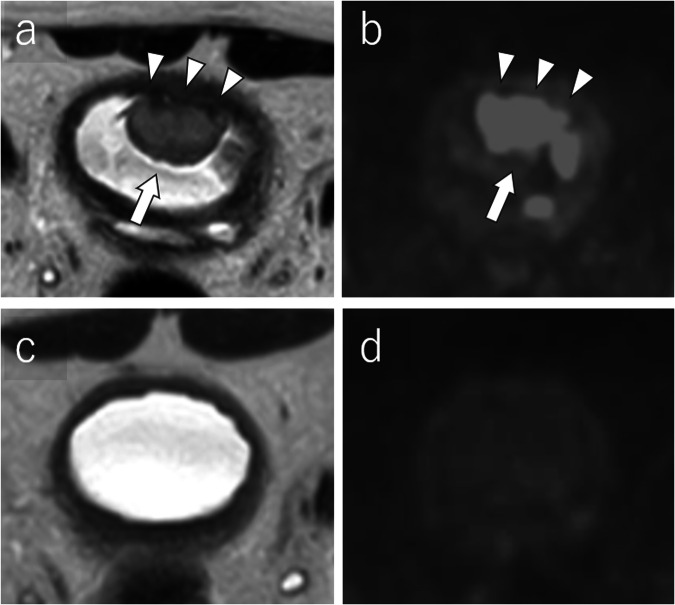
Immunotherapy responder: a 76-year-old man with a 35-mm urothelial carcinoma in the anterior bladder wall pre-immunotherapy MRI shows a round-shaped tumor with no apparent muscularis disruption on T2WI (a) [T2WI VIRADS score: 3] and homogeneous high signal on DW-MRI (b) [DW VI-RADS score: 3]. Post-immunotherapy MRI reveals significant tumor reduction, with no apparent lesions on T2WI (c) or DW-MRI (d). The tumor is indicated by white arrow, and intact muscle layers by white arrowheads. ADC, apparent diffusion coefficient; DCE, dynamic contrast-enhanced; DW, diffusion-weighted; T2WI, T2-weighted imaging; VI-RADS, vesical imaging-reporting and data system
